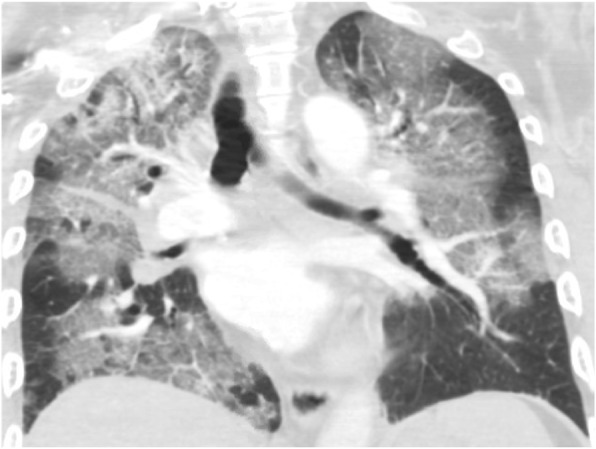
Computed Tomography coronal image through the chest of a patient wih EVALI showing bilateraly patchy ground glass opacities
